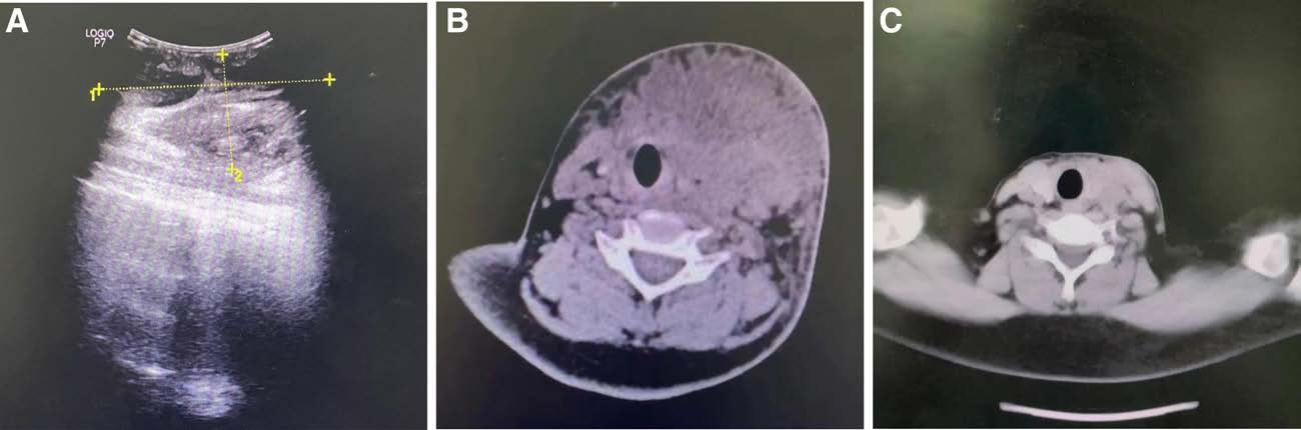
(A) Preoperative ultrasound, (B) preoperative CT, (C) postoperative CT.
